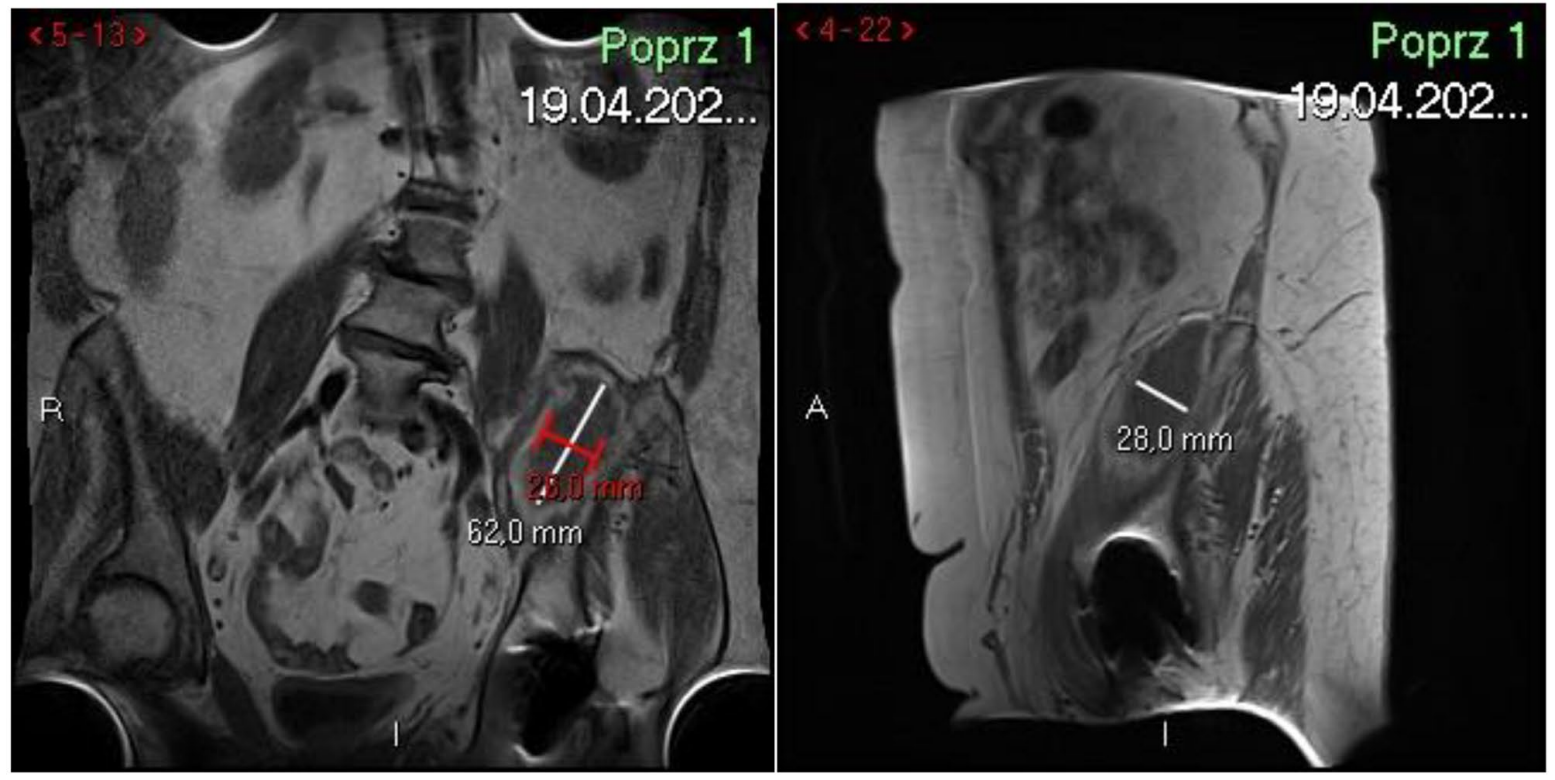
MRI scan with hematoma on the left iliopsoas muscle
